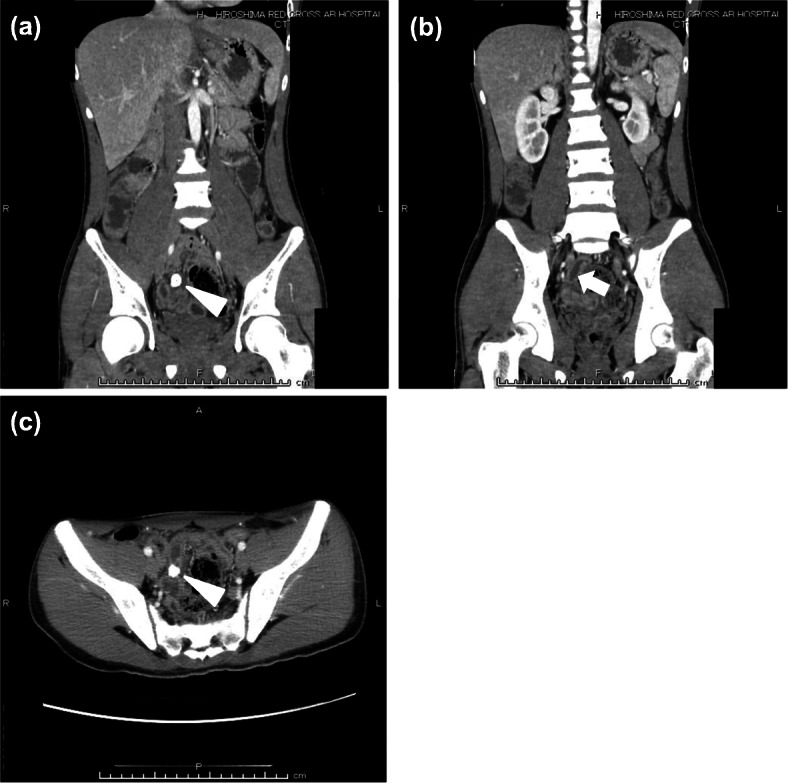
Preoperative enhanced computed tomography (CT) images. Enhanced a, b coronal and c axial CT images showed an enterolith (arrowhead) in a tubular blind-ending structure, with a thickened and hypercaptant wall (arrow)
